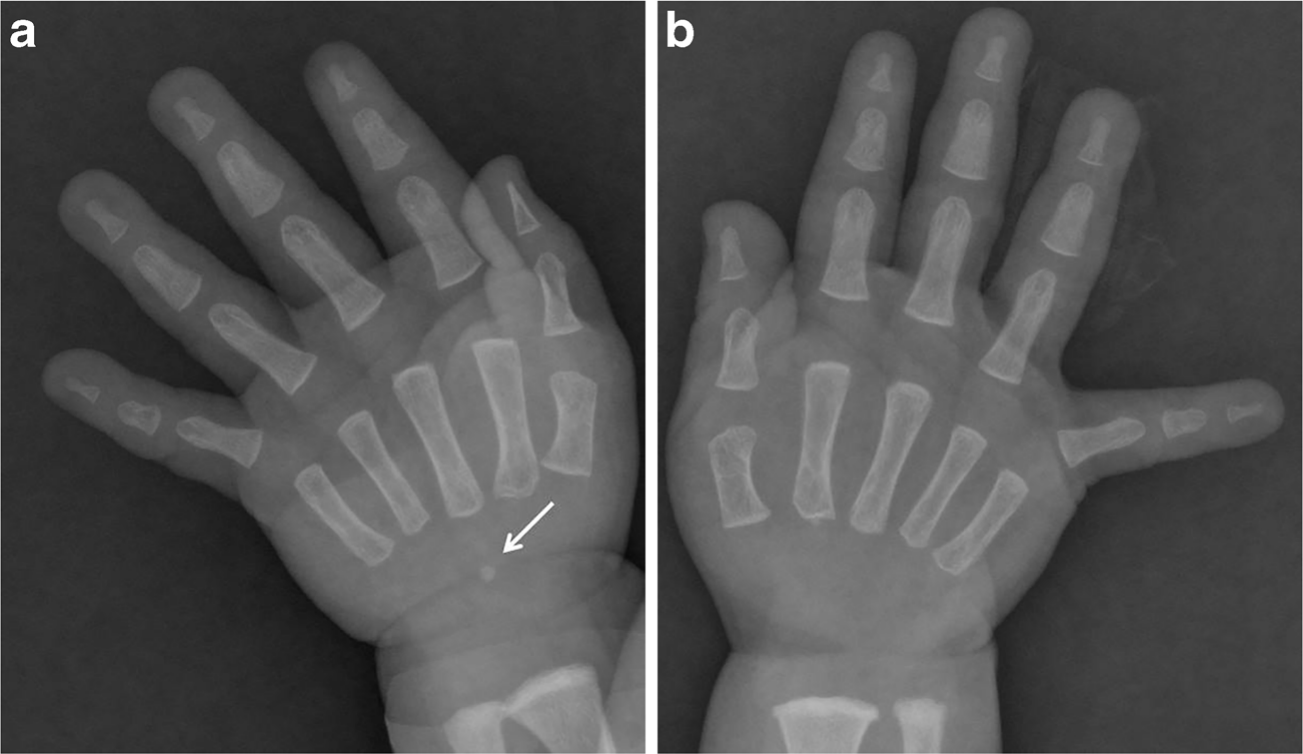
One-month-old boy. (a and b) Left capitate bone (arrow) is demonstrated but right capitate bone and bilateral hamate bones are not demonstrated on the posterior-anterior (PA) radiograph of both hands
